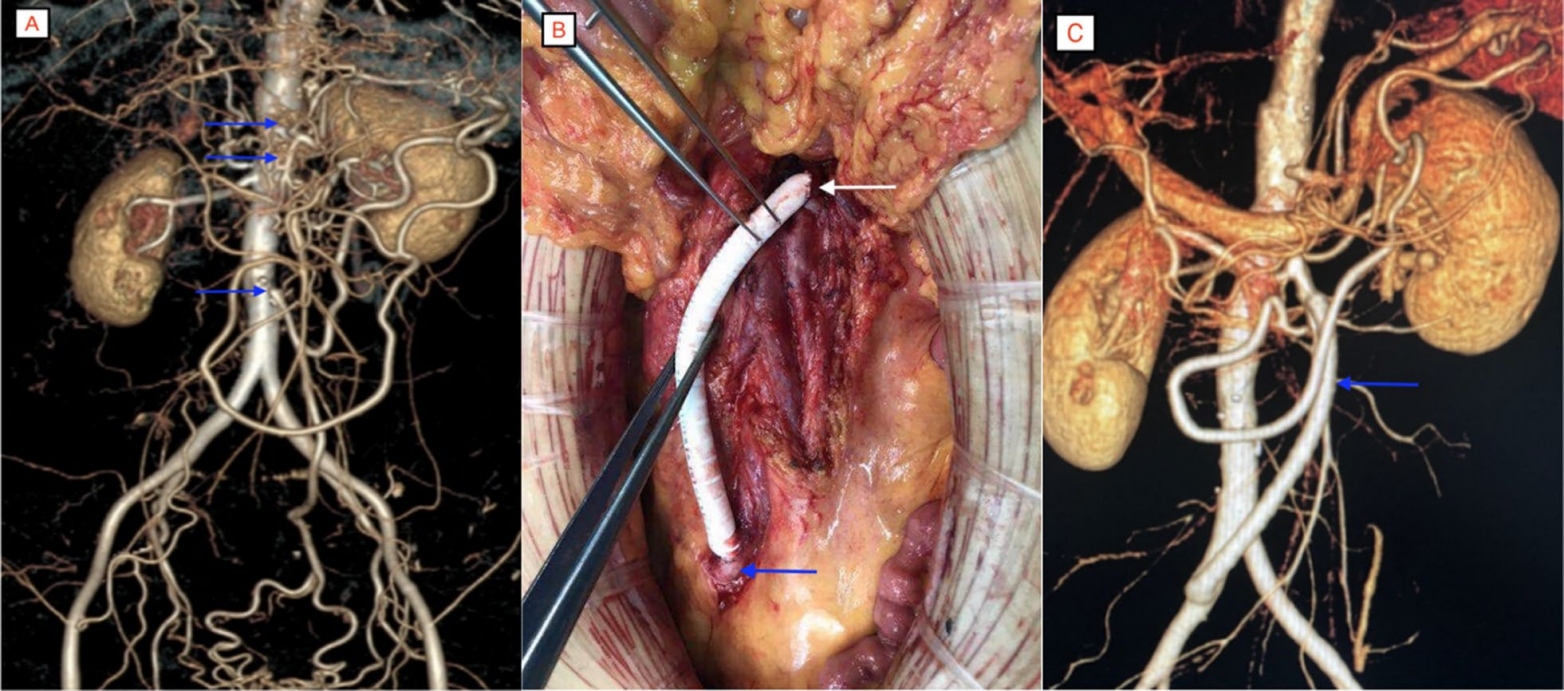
A SMA, CA, and IMA were occluded (blue arrow) with many collateral vessels. B Artificial blood vessel bypass, SMA anastomosis (white arrow), and right common iliac artery anastomosis (blue arrow). C Postoperative CTA image, artificial blood vessel patency (blue arrow)
